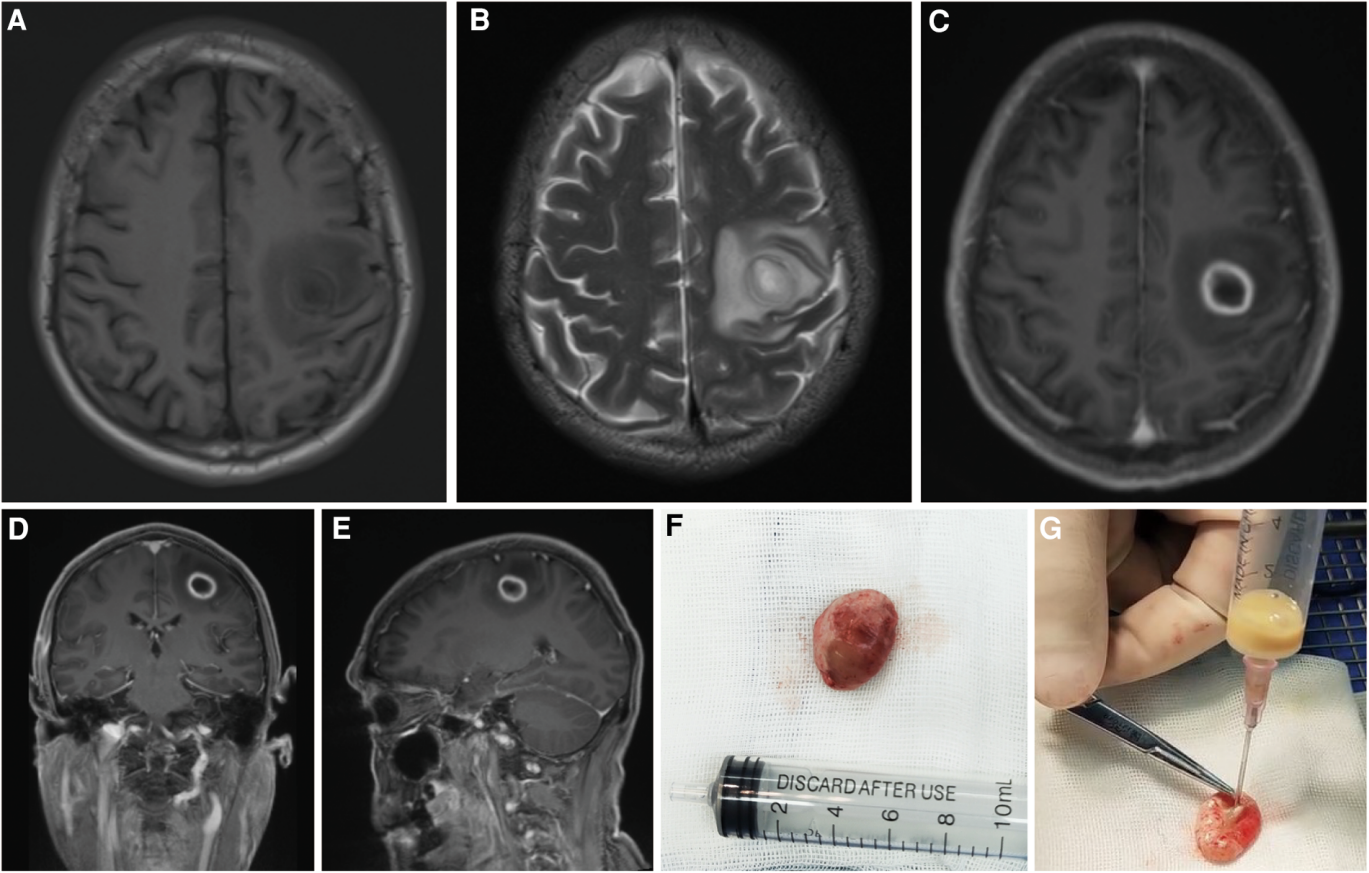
Brain MRI, including axial views in T1WI (A), T2WI (B), and contrast-enhanced T1WI (C), as well as coronal (D) and sagittal (E) views in contrast-enhanced T1WI, revealed the progressive nature of the left frontal lobe lesion. Unbroken cerebral abscess (F) and its pus (G) MRI, magnetic resonance imaging; T1WI, T1-weighted imaging; T2WI, T2-weighted imaging.
